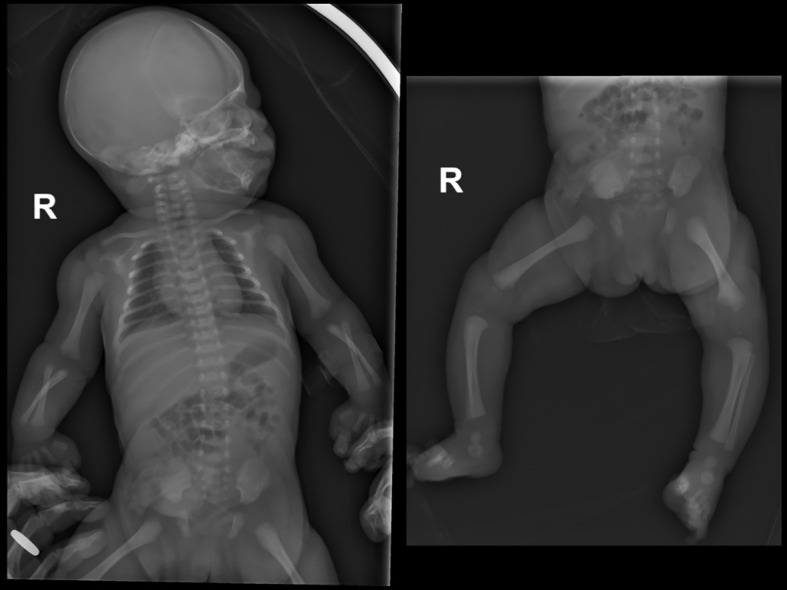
Whole body radiographs of the proband from Family 2 at birth revealed slightly reduced mineralization throughout the entire skeleton.
